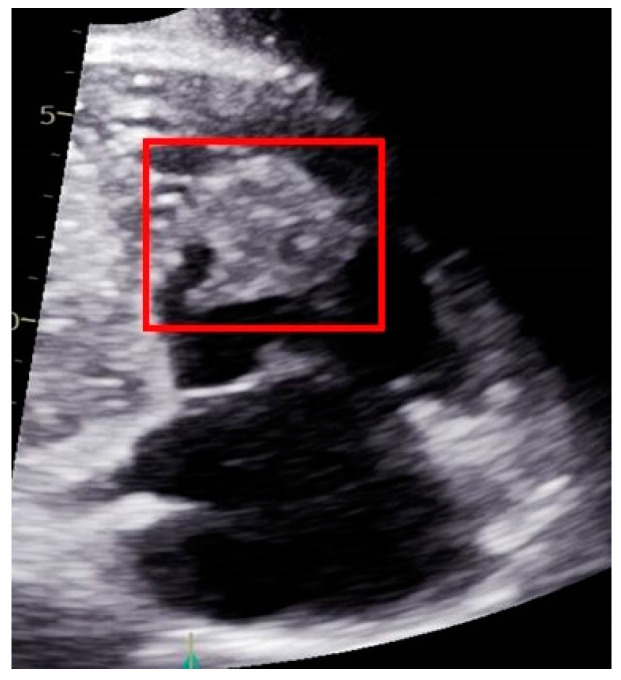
Images from the patient’s echocardiograph showing a cardiac mass in the right ventricle (red square).
